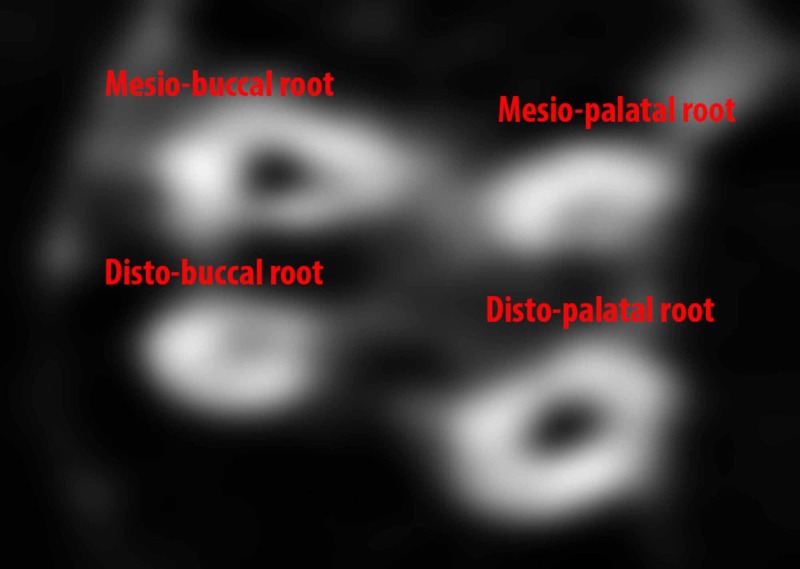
CBCT axial image of #27 CBCT: cone beam computed tomography
